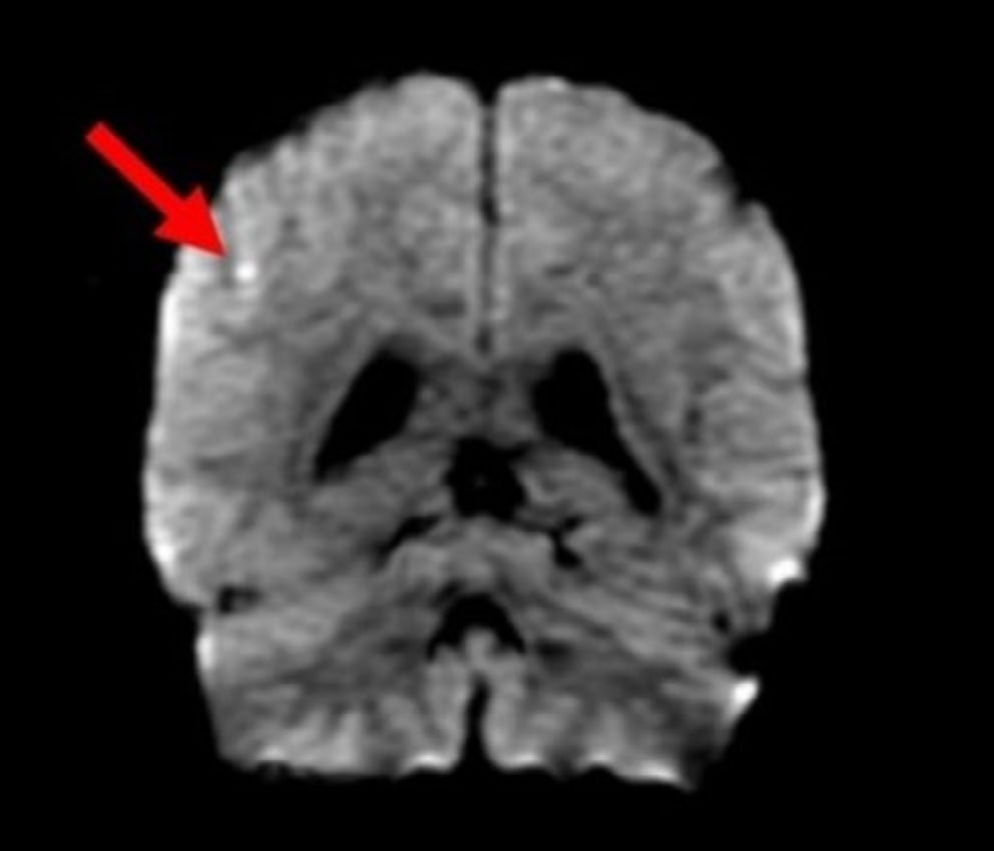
Coronal magnetic resonance imaging of the brain also demonstrating the 3 mm punctate acute ischemic infarct in the right parietal lobe (arrow).
